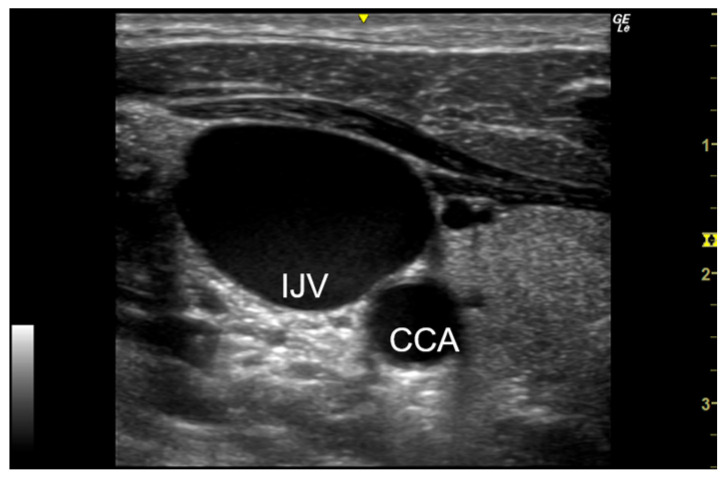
Ultrasonographic images of a normal IJV (transverse section). IJV, internal jugular vein; CCA, common carotid artery.
